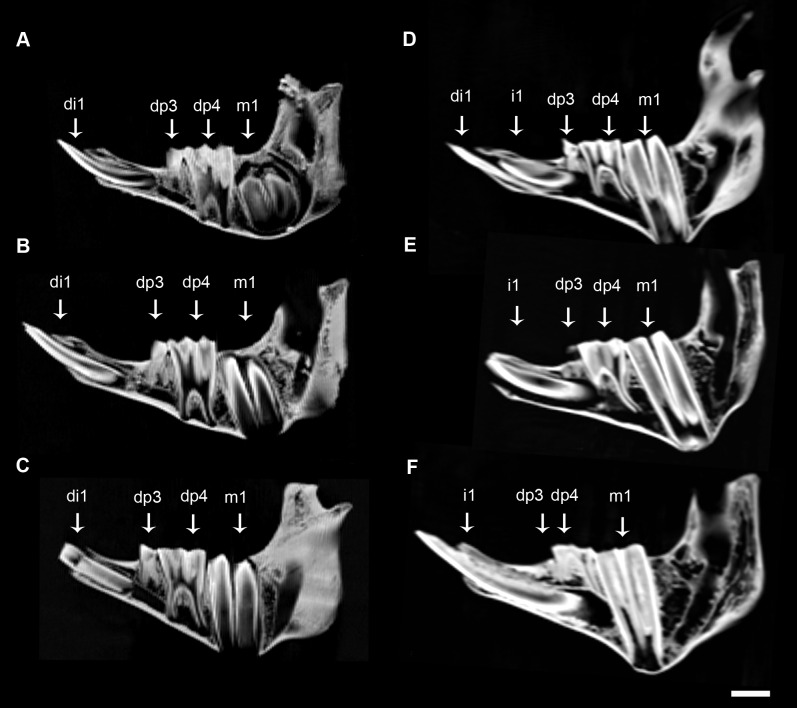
CT-scan images of juvenile lower jaws of *M. balearicus*. Note the development and the replacement pattern of the first incisor. (*A*) MBCN 12592, Cova de Moleta. (*B*) MBCN 12591, Cova de Moleta. (*C*) MBCN 12593, Cova de Moleta. (*D*) IMEDEA 48059, Cova Estreta. (*E*) IMEDEA 85139, Cova des Moro. (*F*) IMEDEA 81013, Cova des Moro. Scale bar  = 1 cm.
